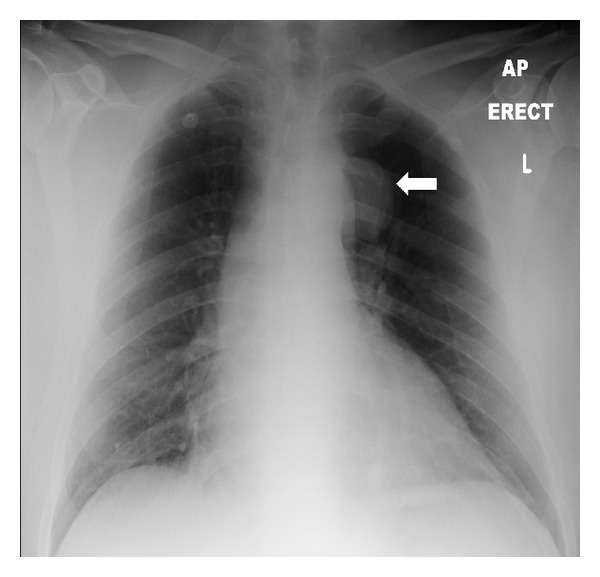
Chest radiograph in a 50-year-old male patient with Castleman disease. A soft tissue pleural-based mass is seen superior to the left hilum (arrow).
